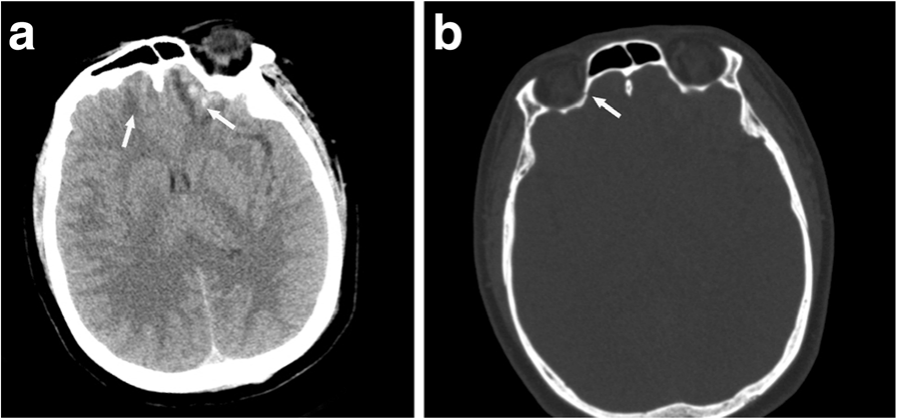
A computed tomography scan revealed a mild bilateral contusion and laceration in the frontal poles (a) and a fracture of the superior portion of the right medial orbital wall (*arrow head*) (b)
